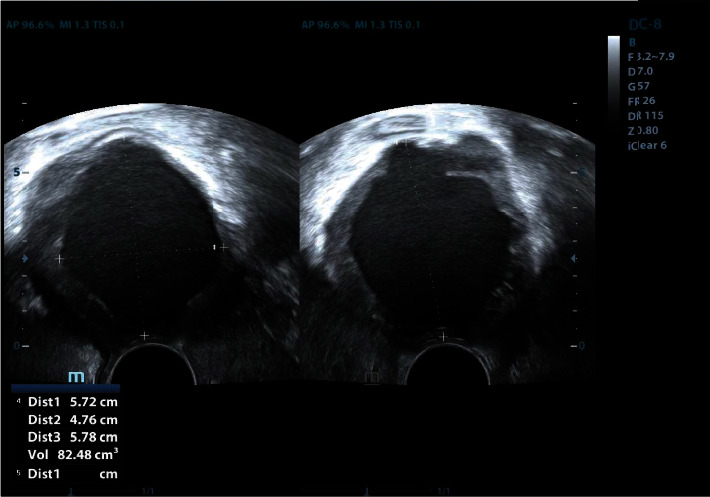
Transvaginal ultrasound image showing a tumor with dimensions of 57.2 × 47.6 × 57.8 mm.
